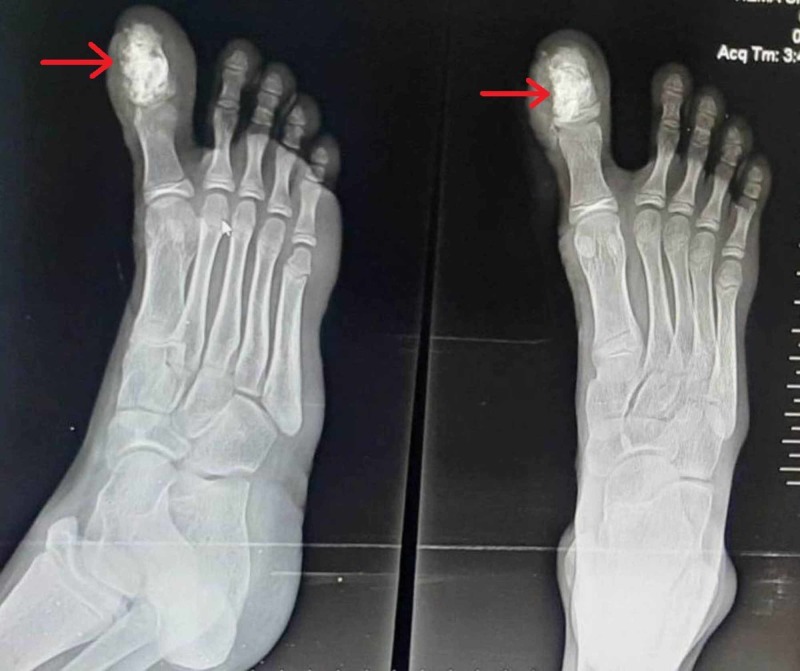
Post operative X-ray of the right foot The image shows defect filled with bone graft
